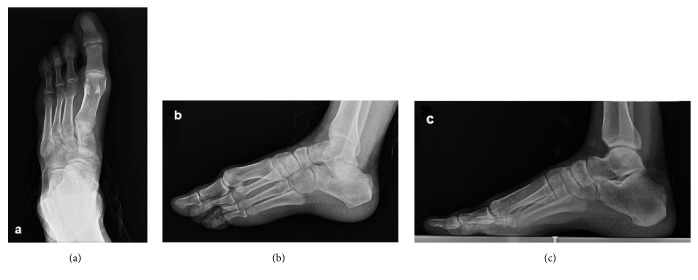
Anteroposterior (a), lateral oblique (b), and mediolateral (c) radiographs of the left foot demonstrate the absence of the fifth finger and the lateral cuneiform. The second to fourth fingers are short. The number, size, and joint relations of the other bones forming the foot are normal.
